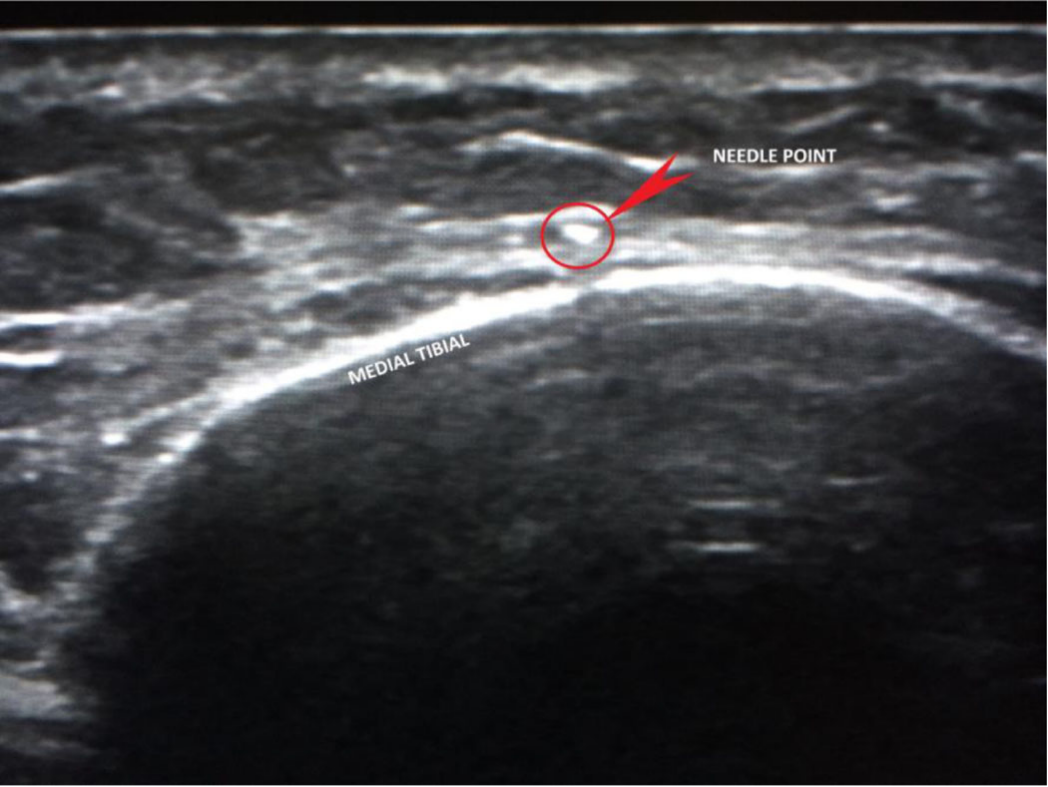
Transverse ultrasound image of the needle position (marked with the red circle/arrow)
